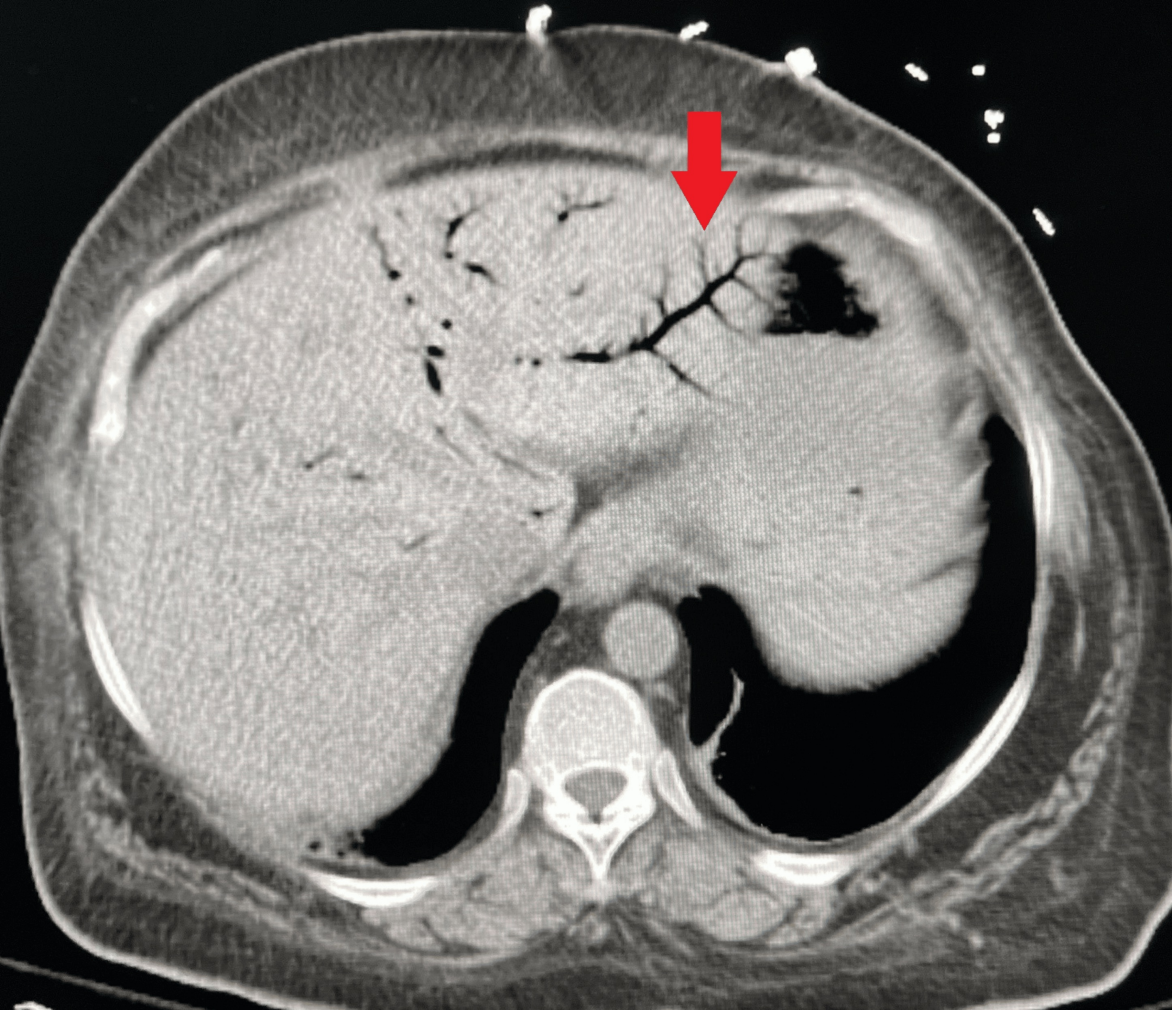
Axial CT of the abdomen demonstrating extensive HPVG, seen as branching hypodensities extending to the periphery of the liver (red arrow). HPVG: hepatic portal venous gas
